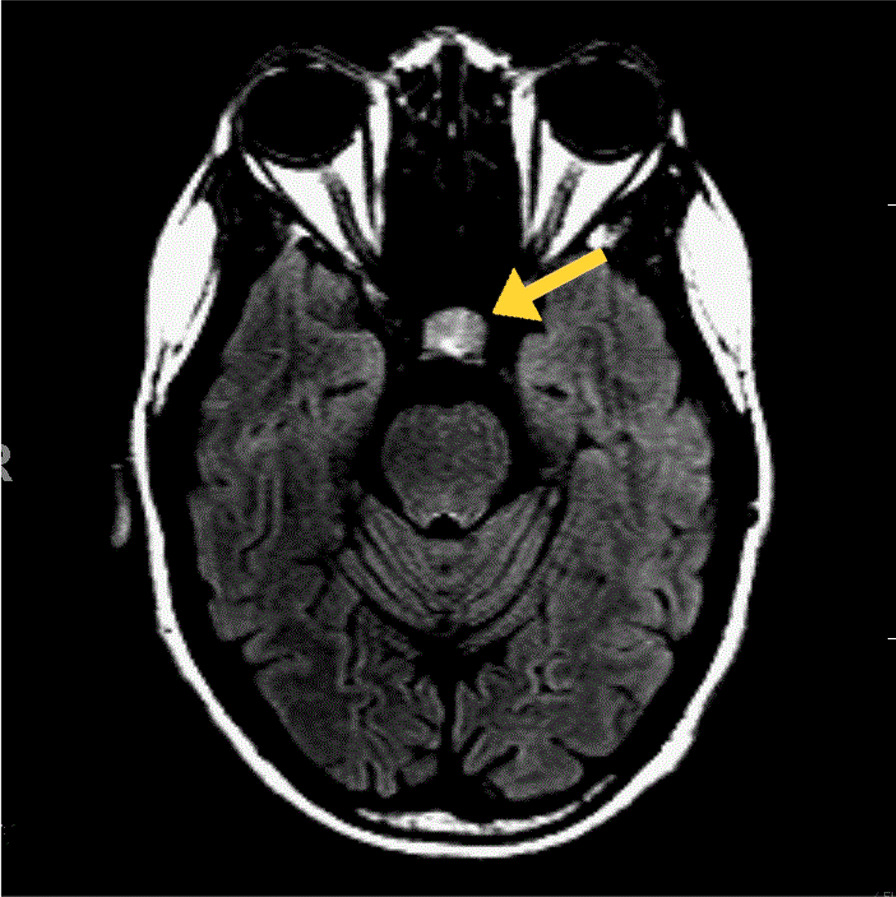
MRI head T2/FLAIR showing a hyperintense pituitary mass, demonstrated by the yellow arrow.
